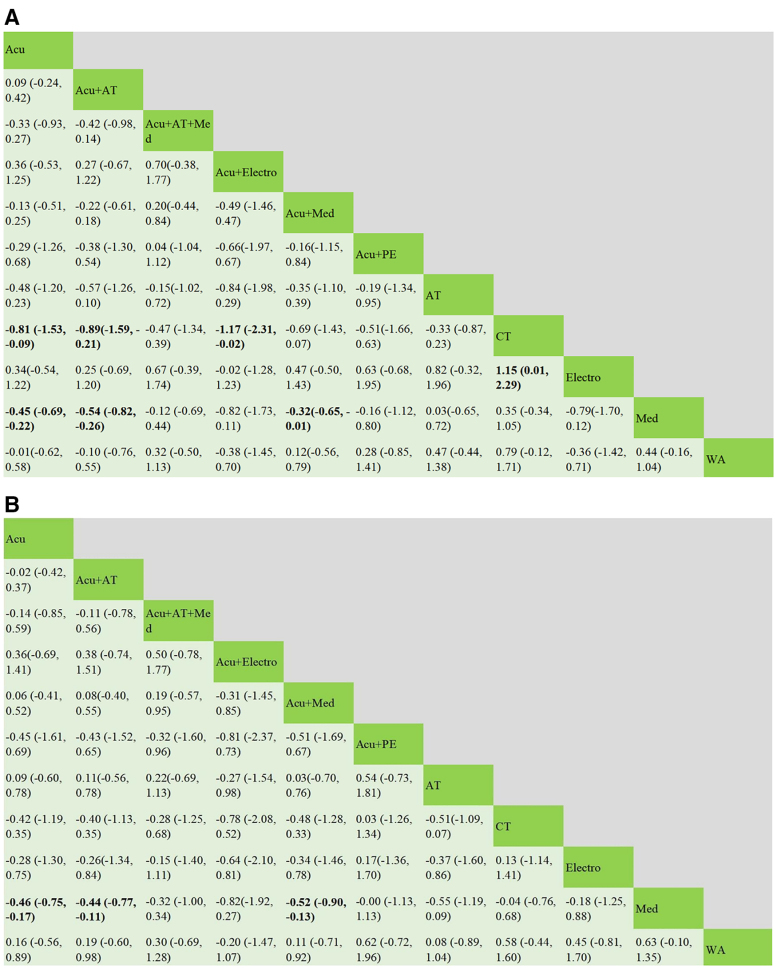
Network meta-analysis results for sleep duration and sleep latency. (A) Sleep duration and (B) sleep latency. Acu = acupuncture, AT = auriculotherapy, CT = conventional treatment, Electro = electroacupuncture, Med = western medicine, PE = point embedding, WA = warm acupuncture.
